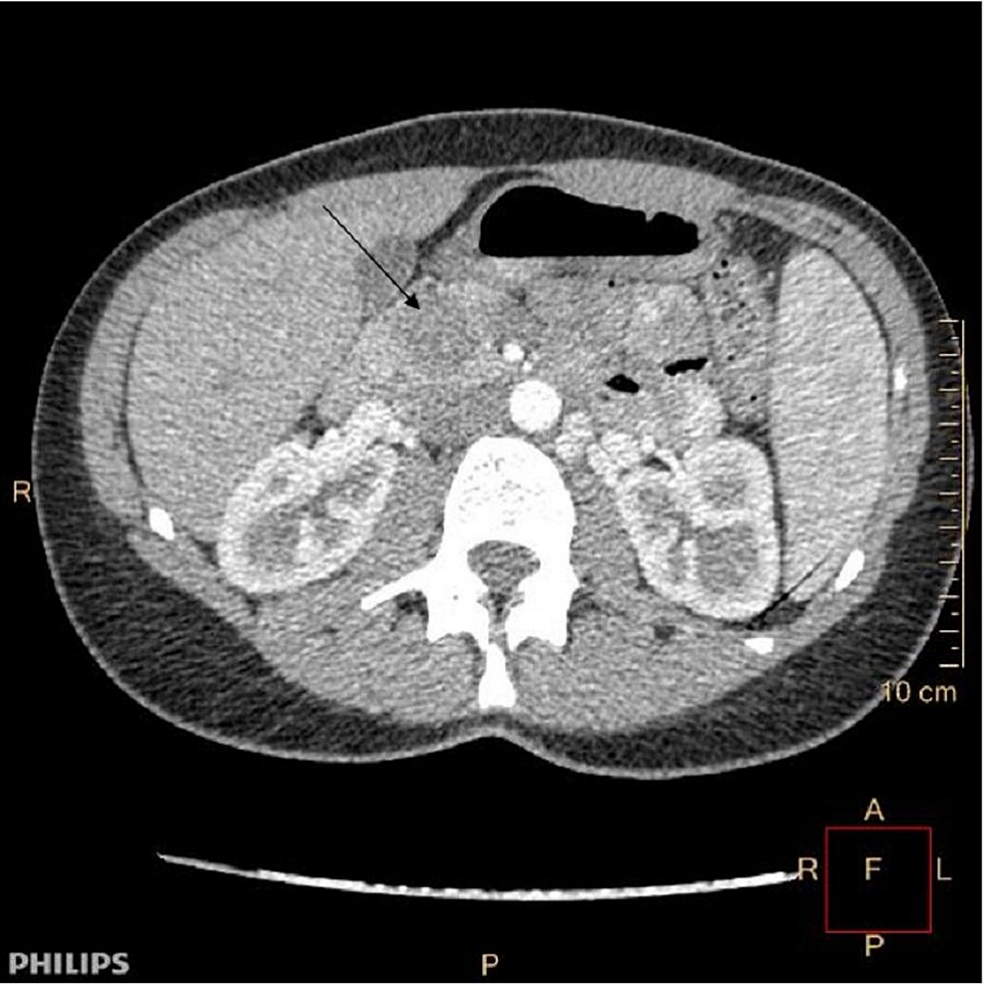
CT scan arrow pointing the pancreatic tumor in the head of the pancreas
